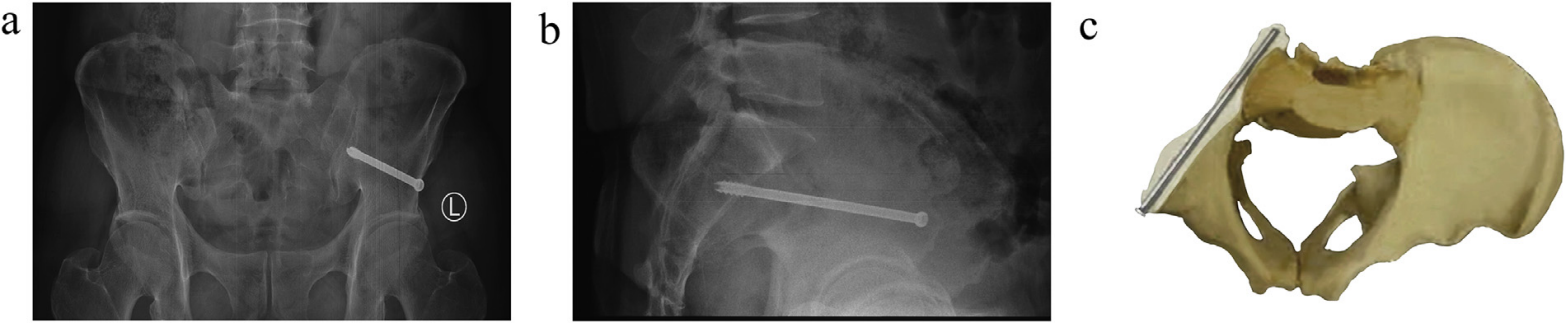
(a) Pelvis X-ray (anterior view). (b) Pelvis X-ray (lateral view). (c) Cross-section view of the model.
